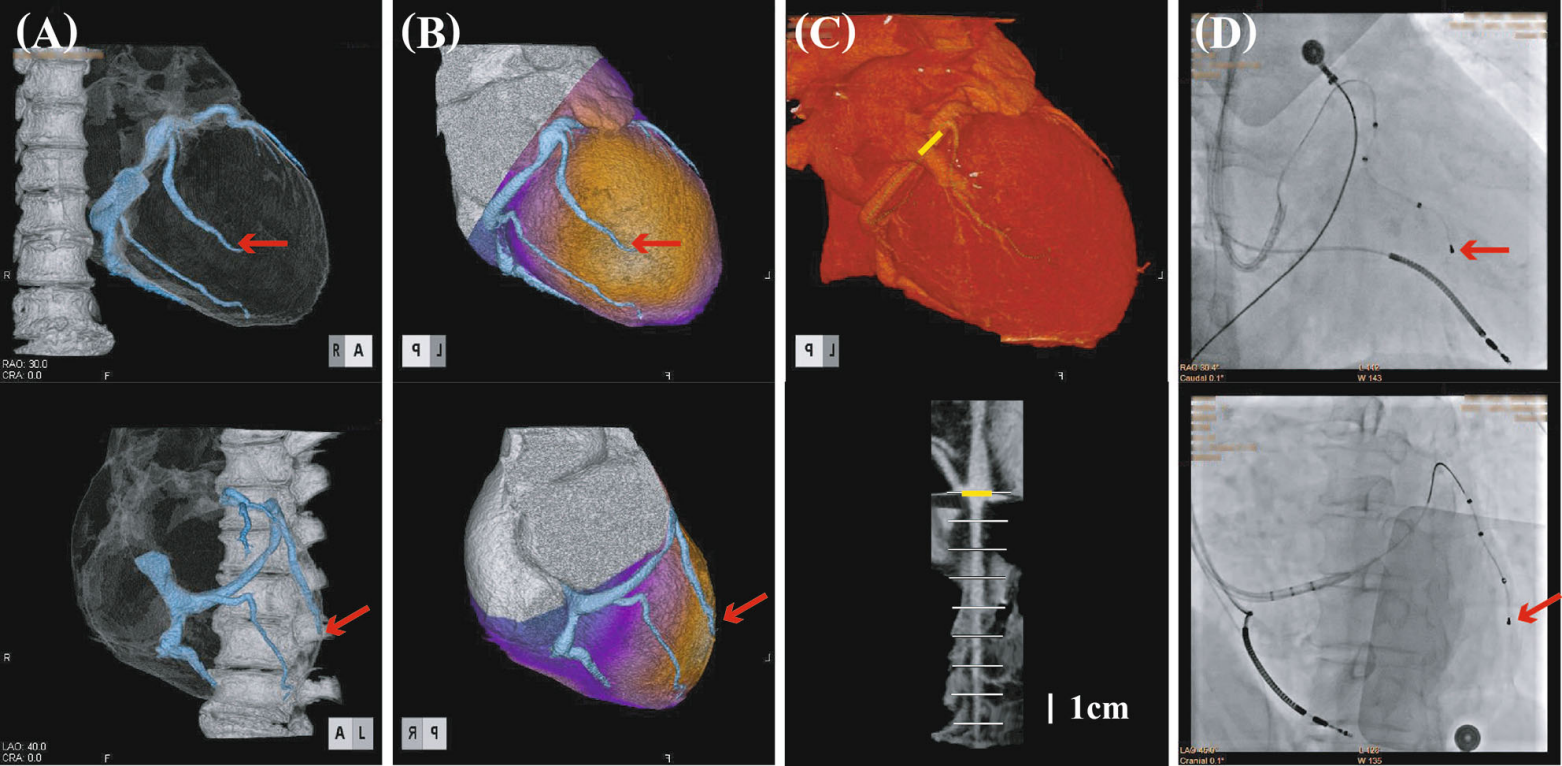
Case 1: A 60-year-old female with idiopathic dilated cardiomyopathy. The vertebral canal and coronary CT venograms are clearly reconstructed (A) then the coronary perfusion image is overlapped (B). The target coronary vein (*red arrows*) is easily selected as an appropriate pacing site in viable myocardium (*yellow areas in B*). Following the measurement of the vein length (C), the CRT pacing lead is appropriately located as planned in advance (*black arrows*, D). Two months later, the end-systolic volume decreased from 188 to 133 mL (a 27% reduction), left ventricular ejection fraction increased from 26 to 31%, and NYHA functional class improved from class III to class II
